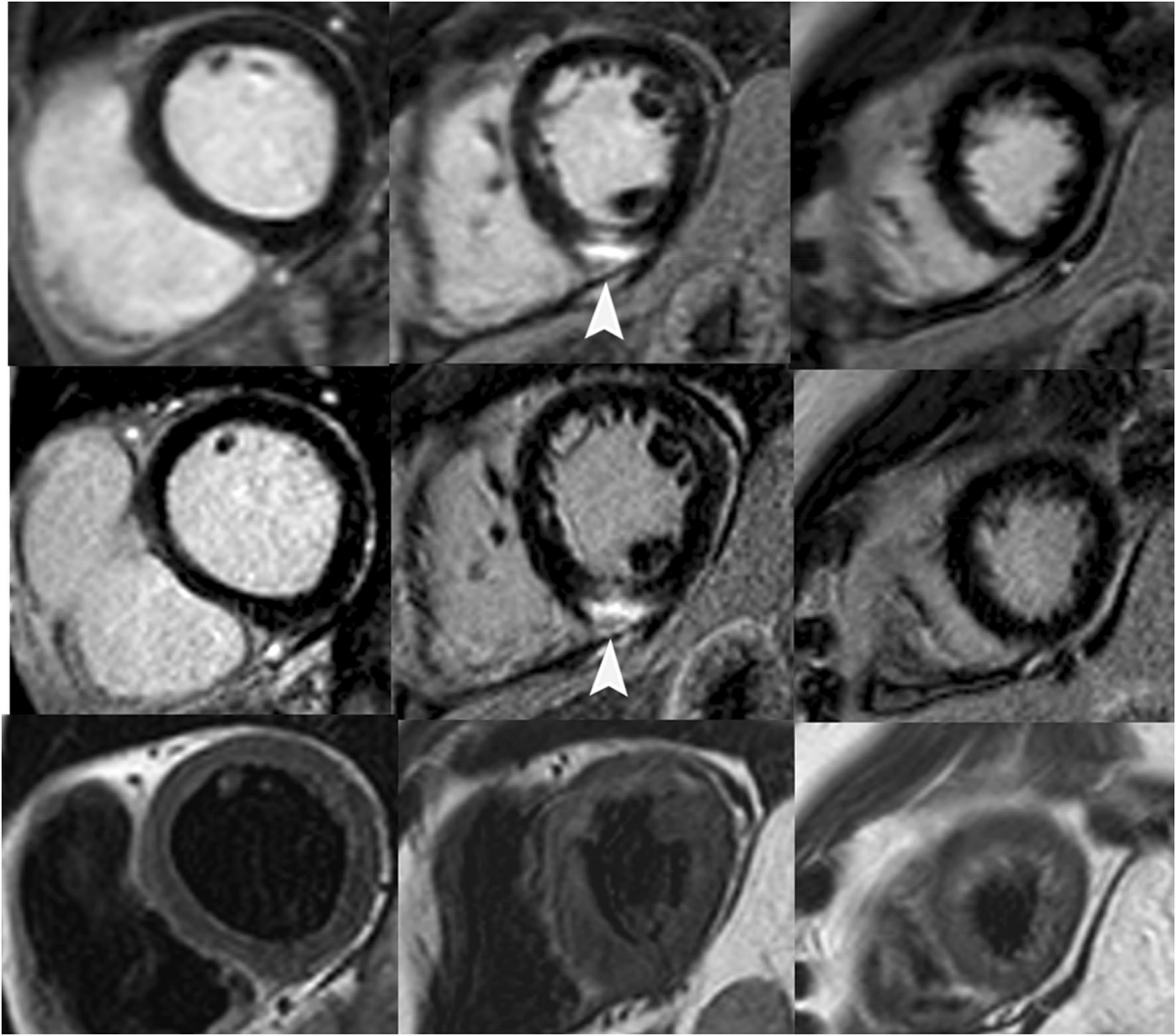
Short-axis LGE CMR images of a 47-year-old male patient with chronic myocarditis. The upper panel shows basal, midventricular and apical sections from 3D LGE acquisition, the middle panel shows the same slices from 2D LGE acquisition and lower panel shows the same slices in a T2 weighted sequence. High signal intensity in LGE images (arrowheads) indicating epicardial fibrous tissue in basal and midventricular inferior segments could be noted in both acquisitions with no signal alterations in T2-weighted images
